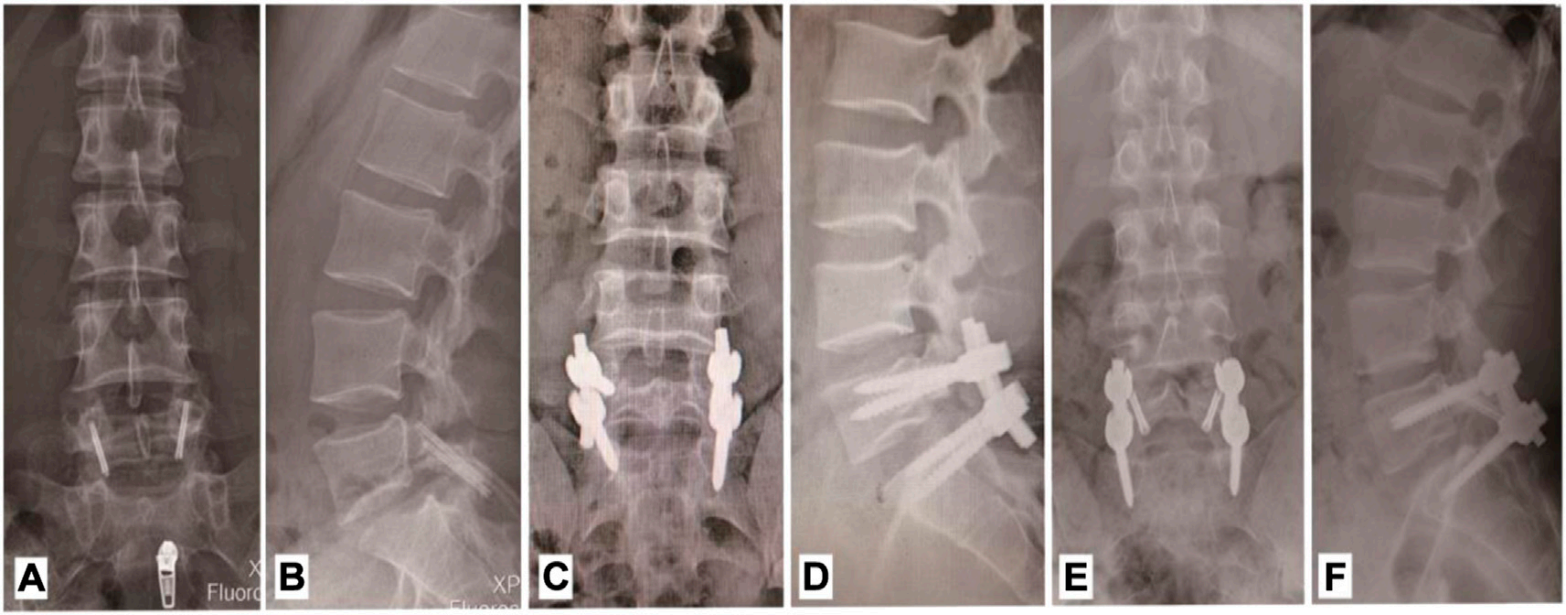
X-ray images of three internal fixation options. (A,B) Anteroposterial-lateral radiograph with intra-segmental direct fixation. (C,D) Anteroposterial-lateral radiograph with intra-segmental direct fixation. (E,F) Anteroposterial-lateral radiograph with hybrid fixation.
